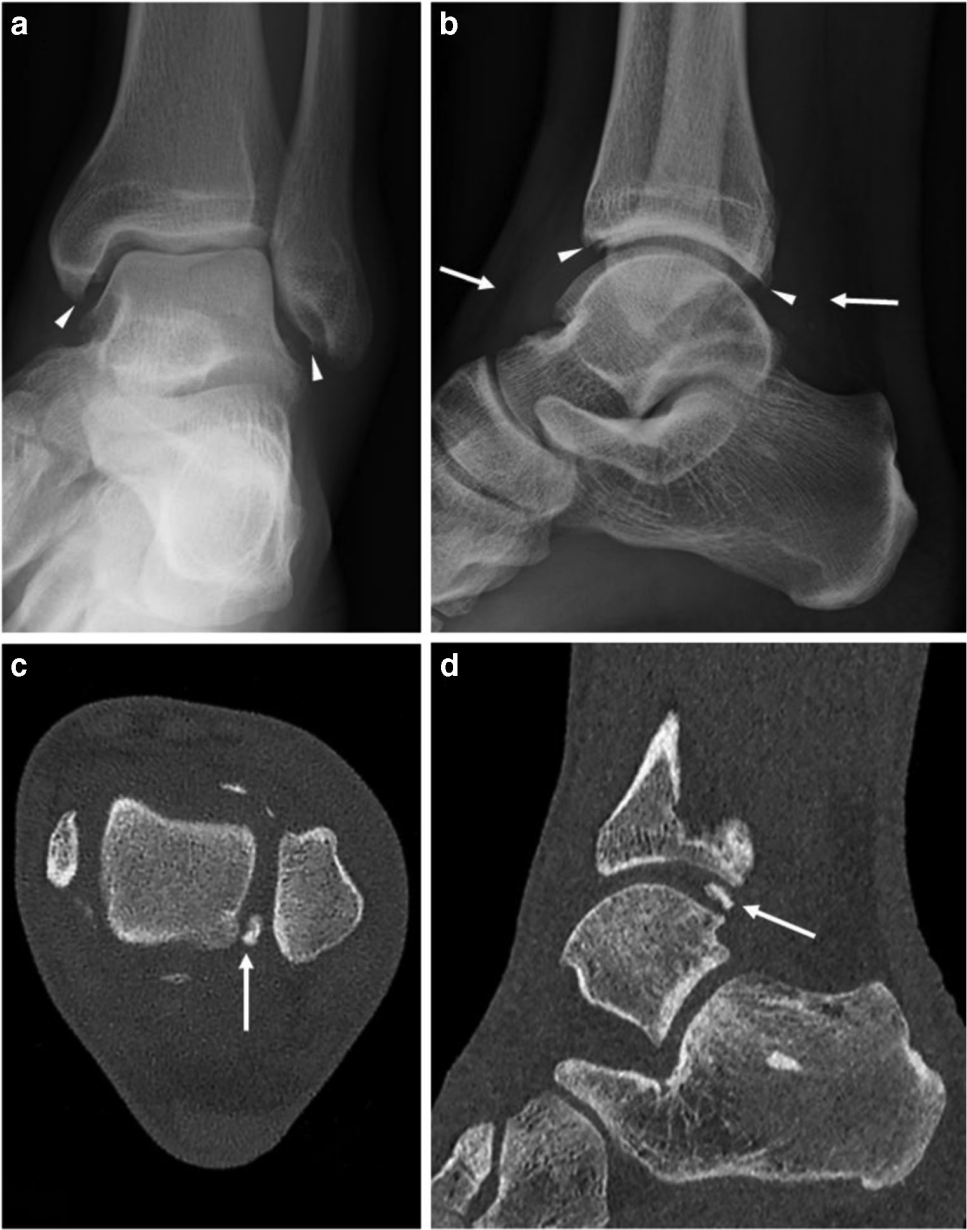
A 31-year-old male that runs several times per week presented to the ER. The same day he experienced a sudden pain in the left ankle and he denies trauma. The left ankle is swollen with limited mobility. The patient is unable to bear weight. Initial DR mortise view and lateral showed signs of early talocrural arthritis with osteophytes surrounding the joint (*arrowheads*, a and b) as well as a joint effusion (*arrows*, b). Subsequent ULD-CT demonstrates a 7-mm loose body in the posterior aspect of the talocrural joint (*arrows*, c and d). The treatment recommendation was upgraded from functional to surgery for removal of the loose intraarticular body
